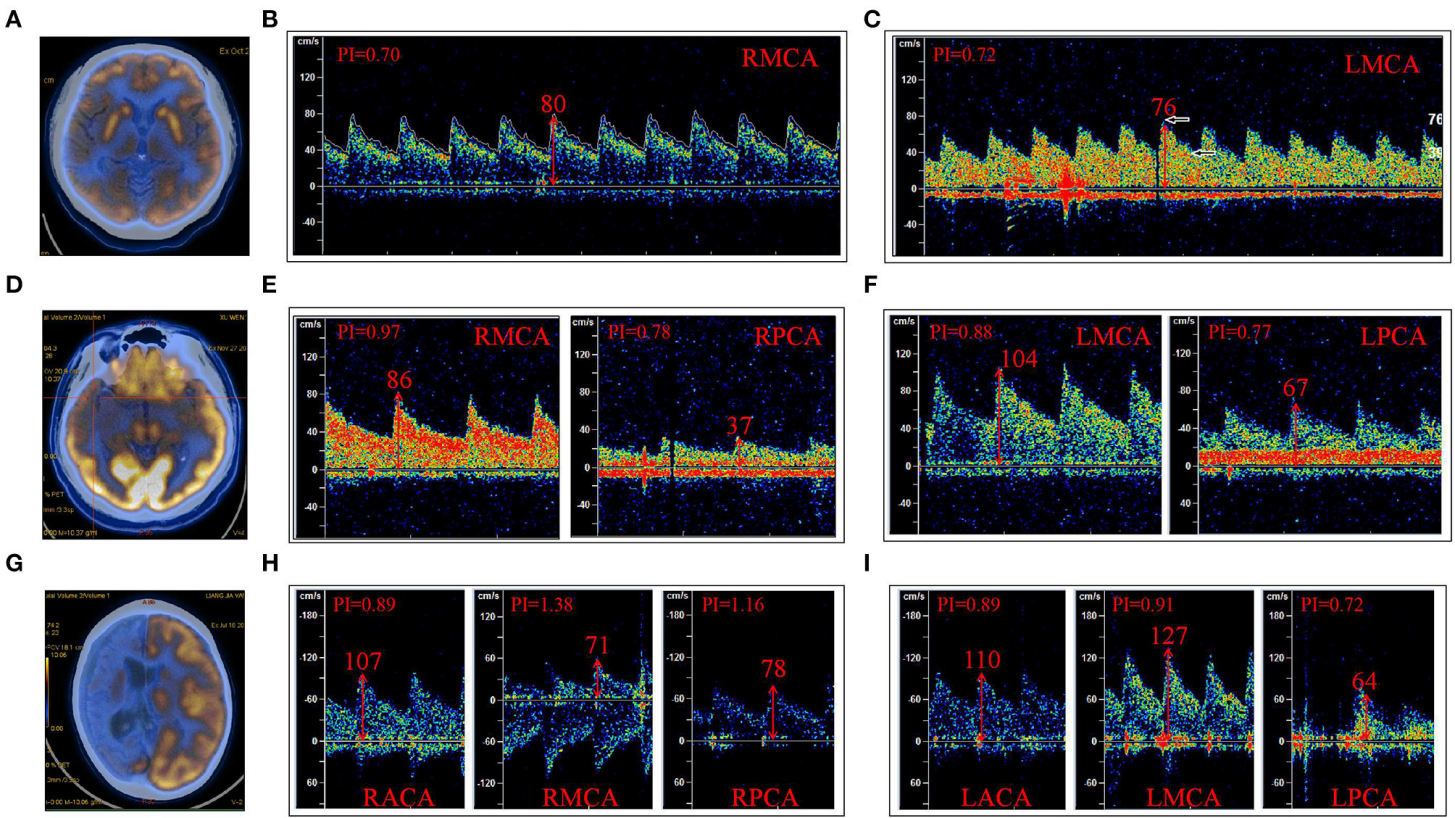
The low metabolic area of FDG^18^-PET-CT (PET) was divided according to the region of the supplying artery. (A) PET indicated a low metabolism in the right frontal and temporal cortex. (B) The PFV of RMCA was 80 cm/s; (C) The PFV of LMCA was 76 cm/s. (D) PET indicated low metabolism in the right temporal lobe and hippocampus; (E) The PFV of RMCA was 86 cm/s, and that of RPCA was 37 cm/s. (F) The PFV of LMCA was 104 cm/s and that of LPCA was 67 cm/s. (G) PET indicated low metabolism in the right cerebral hemisphere. (H) The PFV of RACA, RMCA, and RPCA was 107, 71, and 78 cm/s, respectively. (I) The PFV of LACA was 110 cm/s, LMCA was 127 cm/s, and LPCA was 64 cm/s. (B,C) were case (A); (E,F) were case (D); (H,I) were case (G); R, right; L, left; ACA, anterior cerebral artery; MCA, middle cerebral artery; PCA, posterior cerebral artery.
